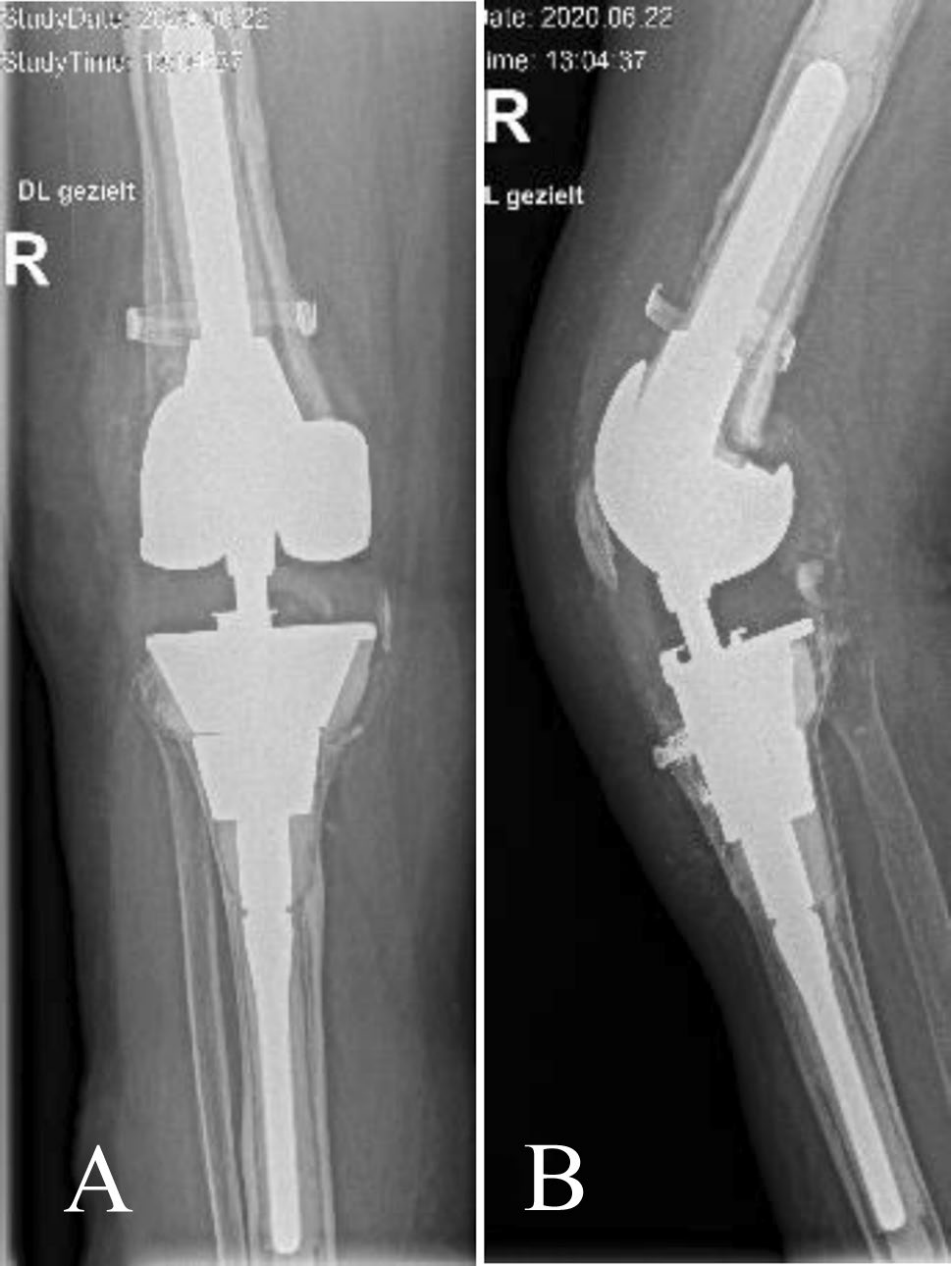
A Right knee, anteroposterior, B right knee, lateral radiographic views after two septic two-stage revisions at time of fifth revision with tantalum metal cones (TMC). In this knee, both components are loose with double cone technique at tibia, prophylactic cerclage at femur, cemented stems, fixation of the tibial tuberosity osteotomy with two screws into the TMC and complete radiolucent lines at 8.2-year follow-up (FU) (patient who denied re-revision surgery and was calculated as septic failure)
